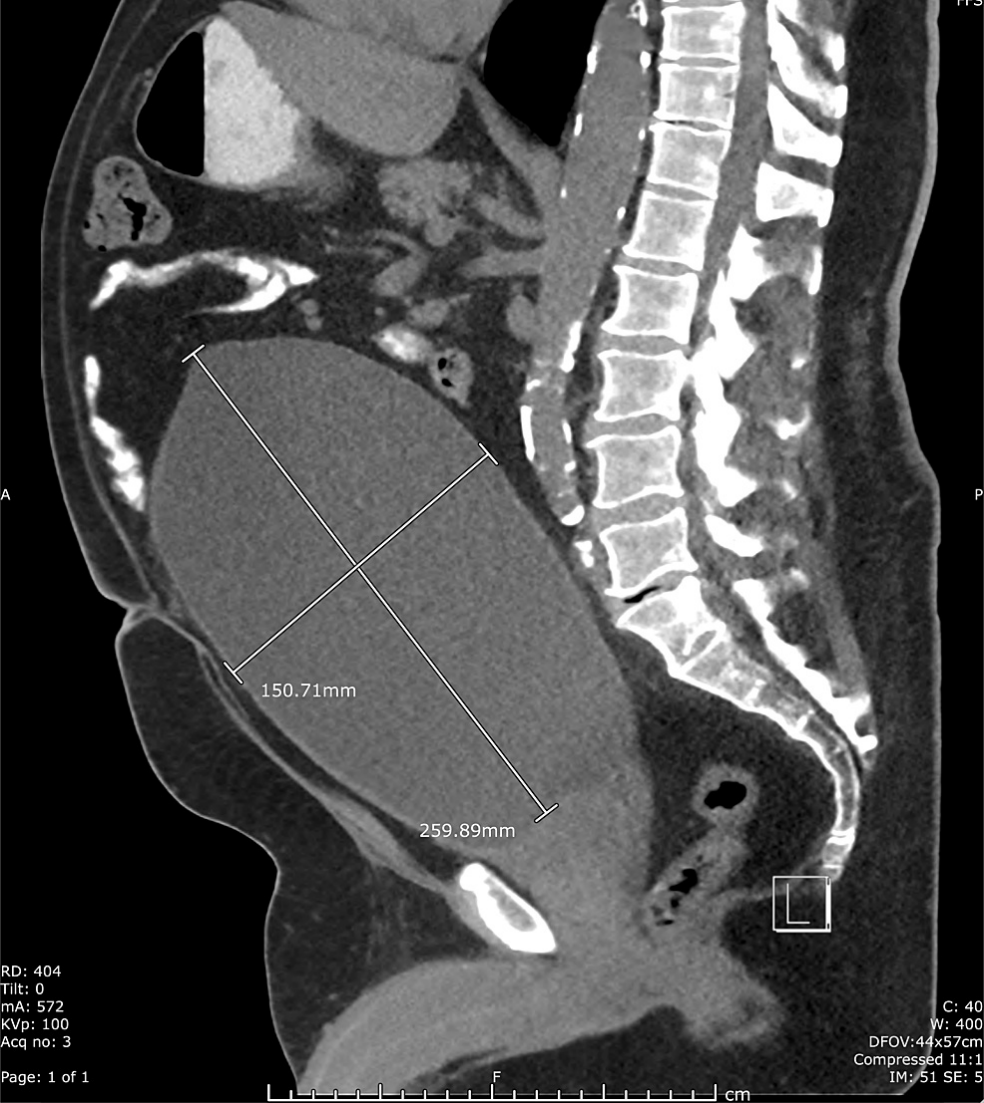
Sagittal view of the CT abdomen and pelvis with severe urinary bladder distention. The urinary bladder measures 259.89 mm by 150.71 mm.
